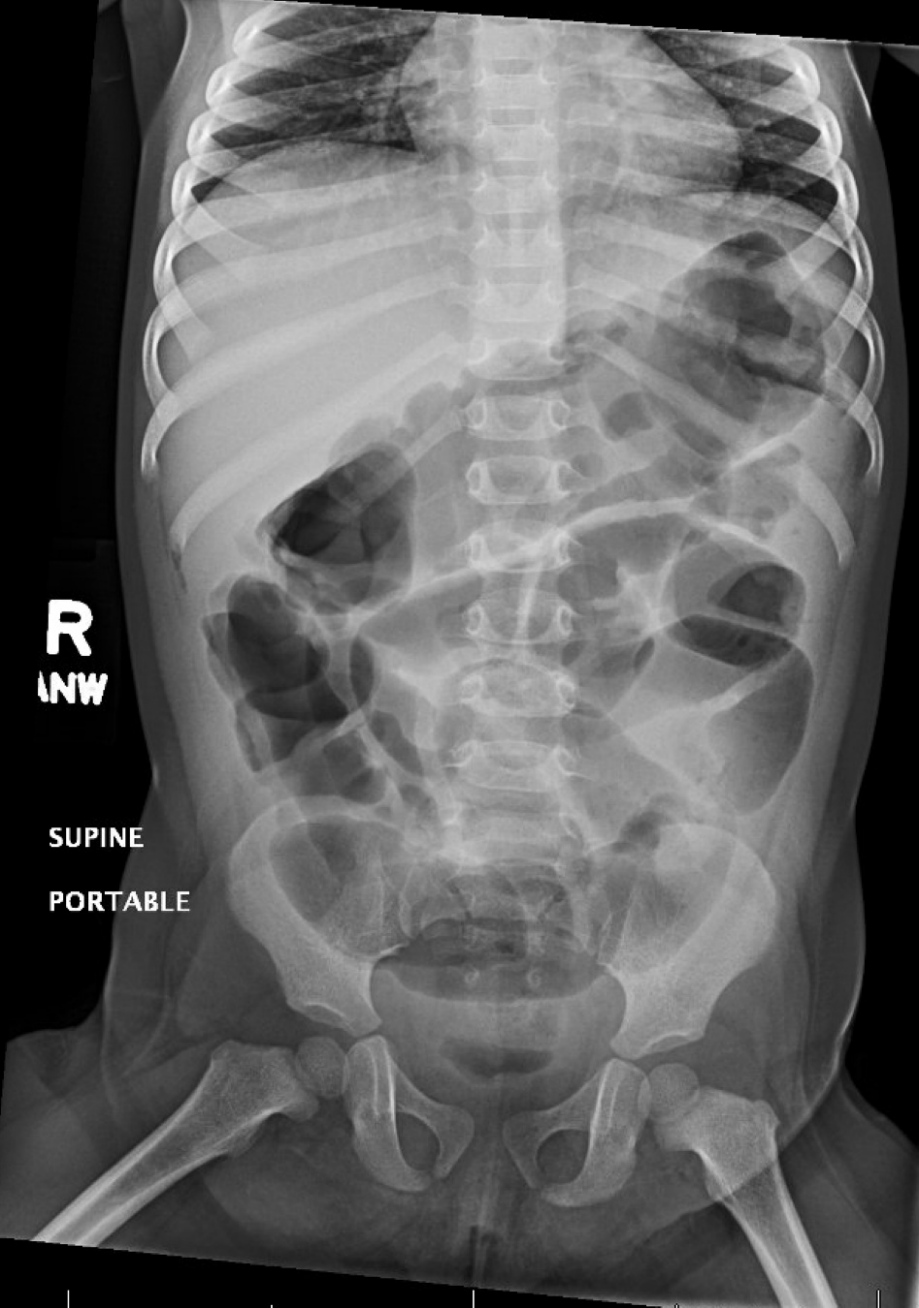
Initial radiograph on admission demonstrating non-obstructive gas patterns without evidence of any discrete masses or abnormal calcifications
